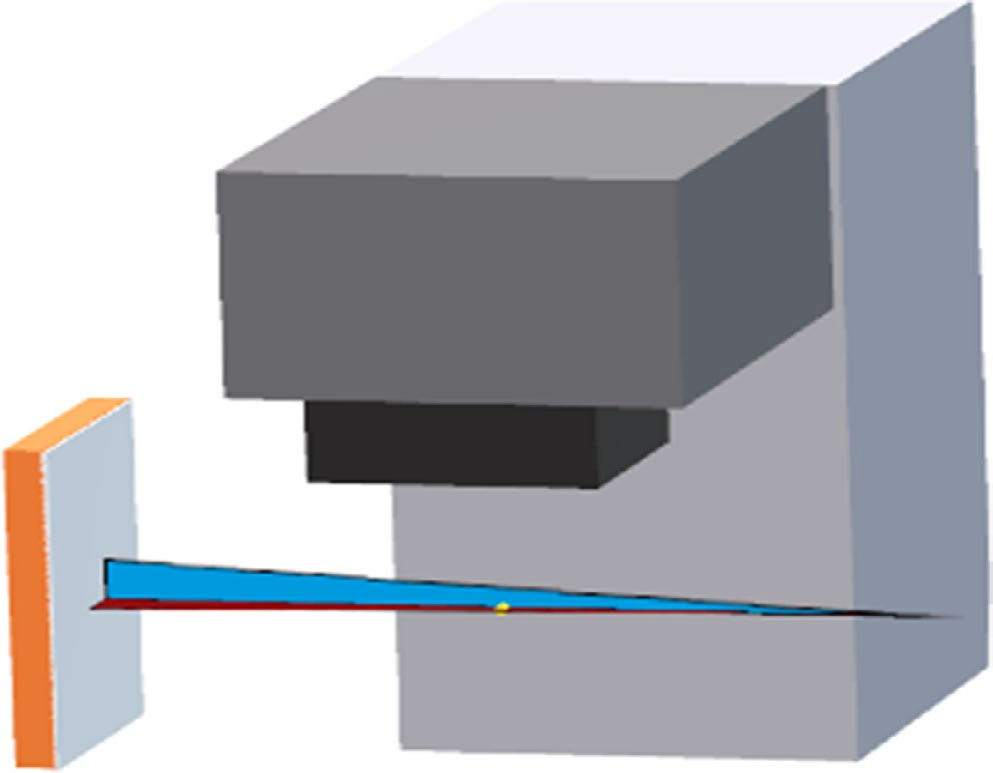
3D coordinates of the fiducials into the 2D planar kV imager coordinate system using a cone‐beam geometry at the linac
